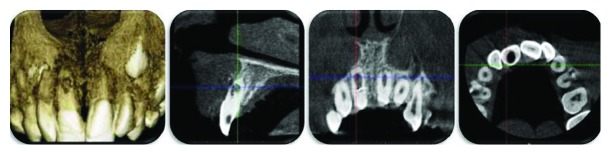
Pretreatment CBCT images.
